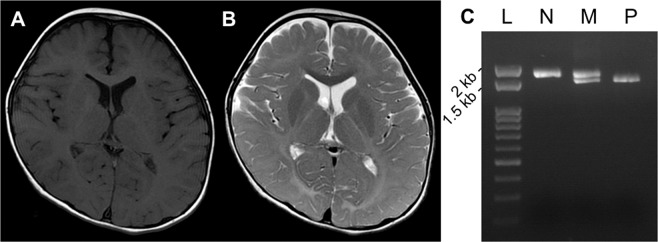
Information of the present patient. T1- (A) and T2- (B) weighted brain magnetic resonance imaging examined at 2 years of age shows T2-high intensity in the deep white matter. A normal myelination pattern is shown in the corpus callosum. There is no structural abnormality. C Genomic PCR for exons 3–4 of *PLP1*. In comparison with the normal control (N), an abnormal short band is shown in the patient (P). The mother (M) exhibits both bands, indicating that she is a heterozygous carrier of this variant. L; Gene Ladder 100 (Nippon Gene, Tokyo, Japan).
